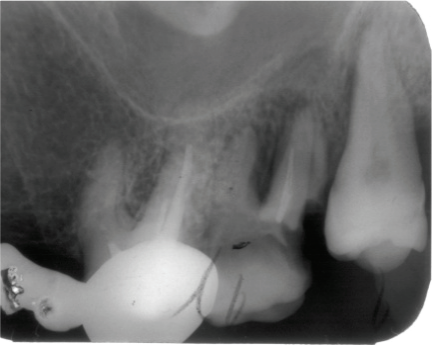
Preoperative radiograph indicates bone loss around the apices of 27 and furcal radiotransparency 26 and 27
